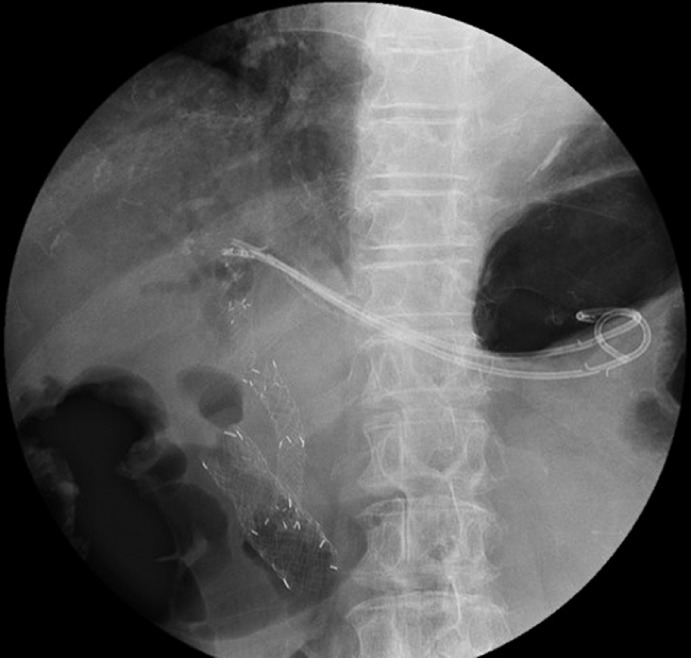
During a second endoscopic reintervention for self-expandable metal stent occlusion, new plastic stents were placed through the distal end of the endoscopic ultrasound-guided hepatogastrostomy (EUS-HGS) self-expandable metal stent (SEMS), after the stent mesh had been broken by argon plasma coagulation, because of the difficulty removing the EUS-HGS SEMS.
